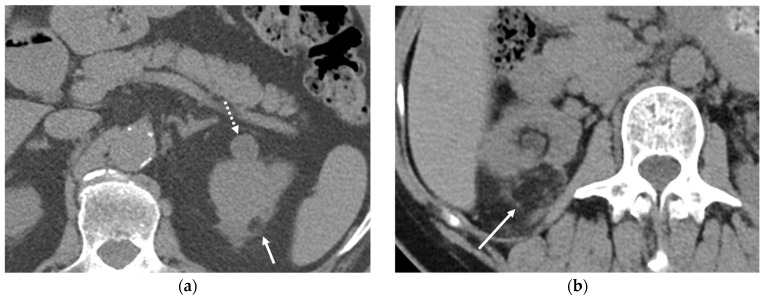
Incidental renal findings in low-dose computed tomography (LDCT) scans. (a) An axial LDCT scan of a 67-year-old male demonstrating a 9 mm fat-containing nodule (arrow) in the left kidney, suggesting angiomyolipoma. But the incidental finding of the soft tissue nodule (dashed arrow) warrants further evaluation. (b) An axial LDCT scan of a 49-year-old female showing an exophytic right upper renal angiomyolipoma (arrow) measuring 4.6 cm in diameter, which may be associated with a risk of spontaneous rupture.
